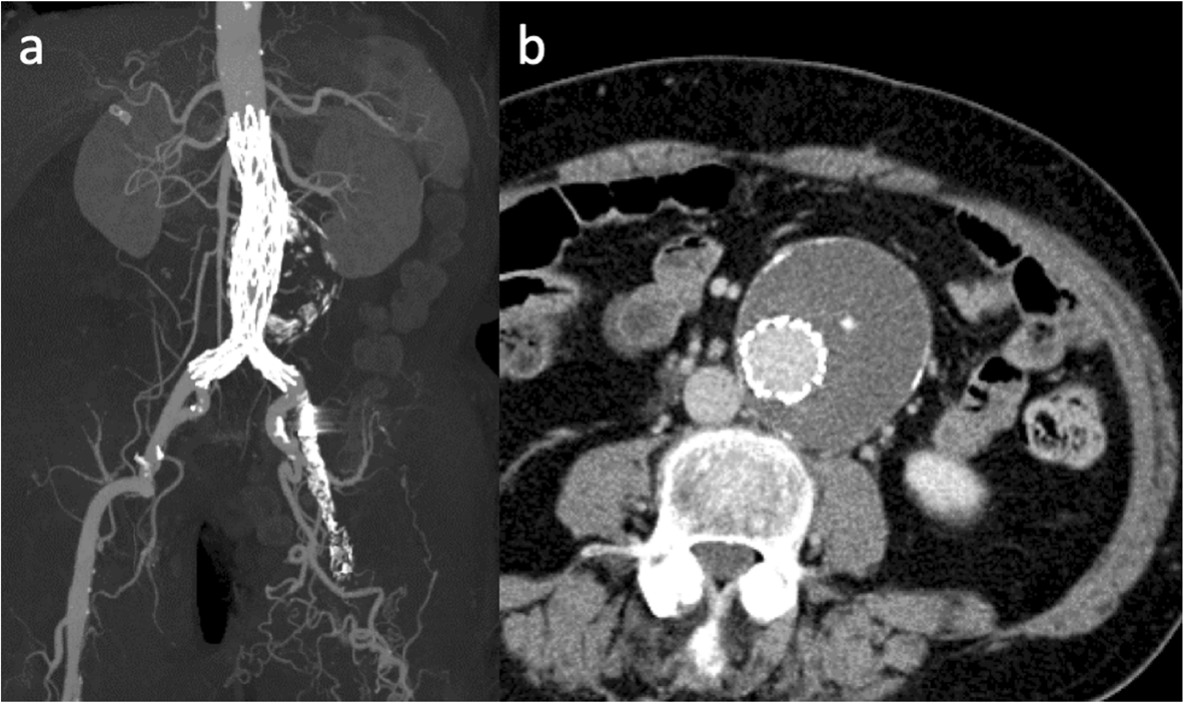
Post operative CT. a: MIP image of CT angiography shows an implanted stentgraft in the aorta with the bilateral legs deployed in the CIA, b: AAA was successfully sealed without any visible endoleak. AAA: abdominal aortic aneurysm, CIA: common iliac artery, MIP: maximum intensity projection
